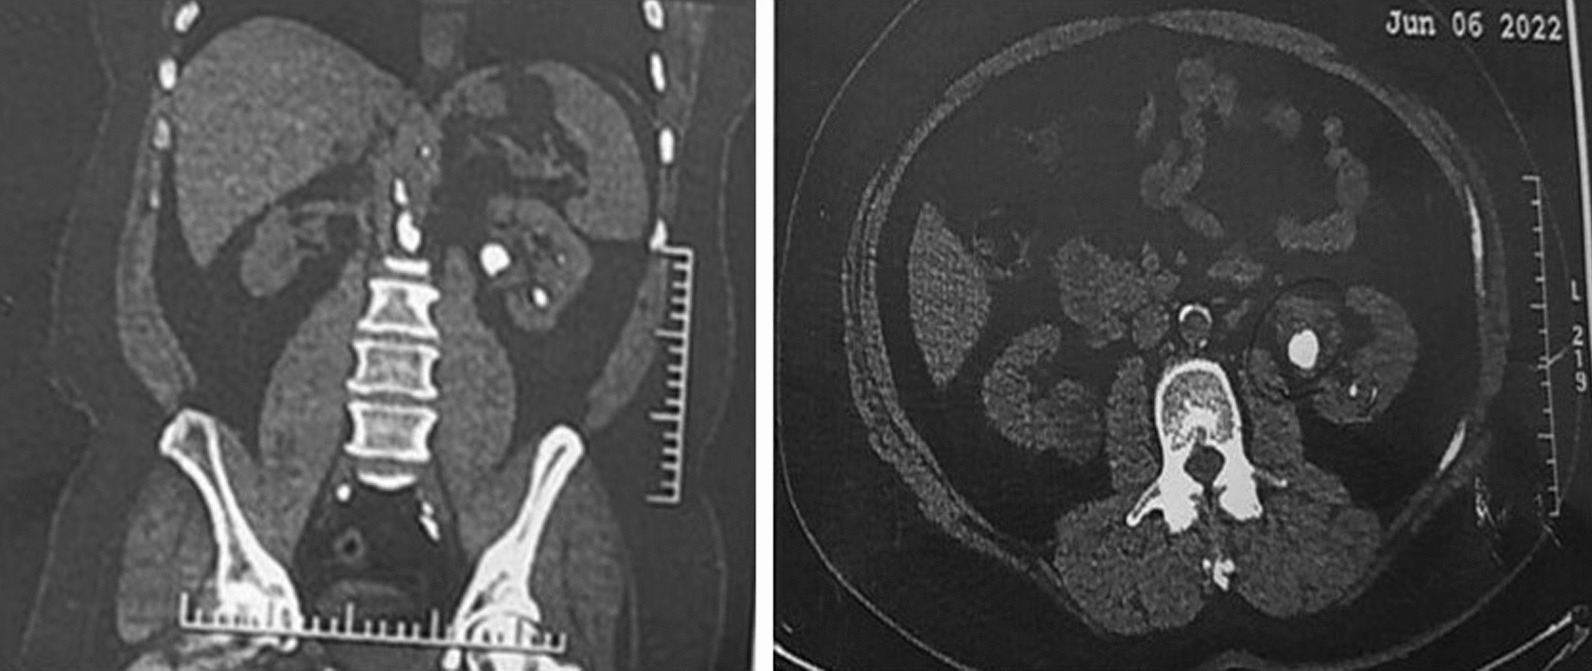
Computed tomography scan of the first case indicating urinary stones in the left kidney
